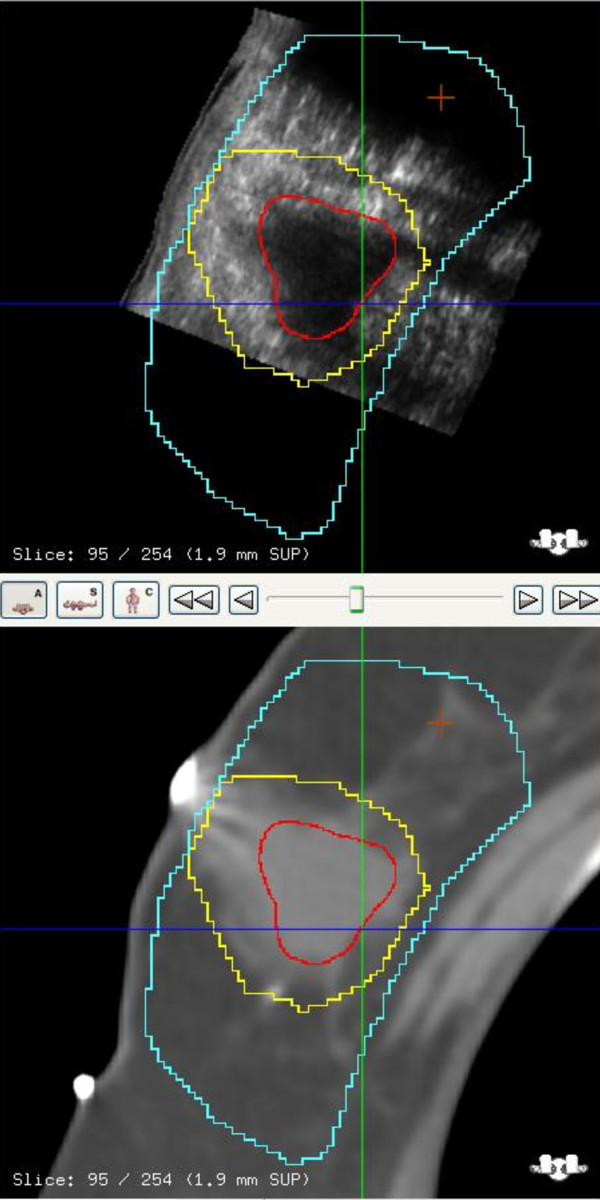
Axial view of a fused CT/3D-US dataset. The ultrasound-based reference volume (RV) is in red, the CT-based GTV in yellow, and the PTV in blue.
